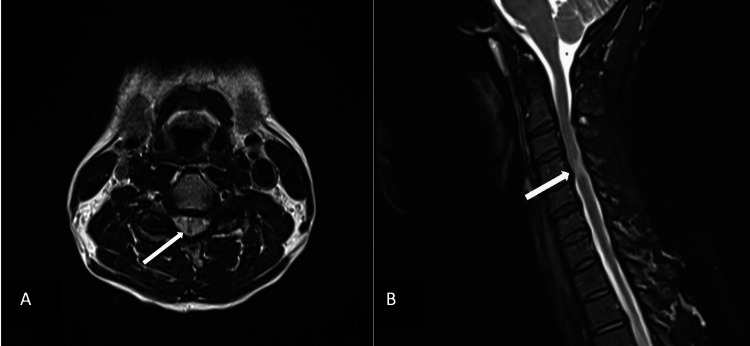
Axial (A) T2-weighted MRI of the cervical spine showing symmetric bilateral lateral cord signal abnormality at C4-C5. Sagittal (B) T2-weighted MRI of the cervical spine with neutral neck position showing cord signal abnormality at C4-C5 with cord atrophy at that level without obvious extrinsic cord compression.
